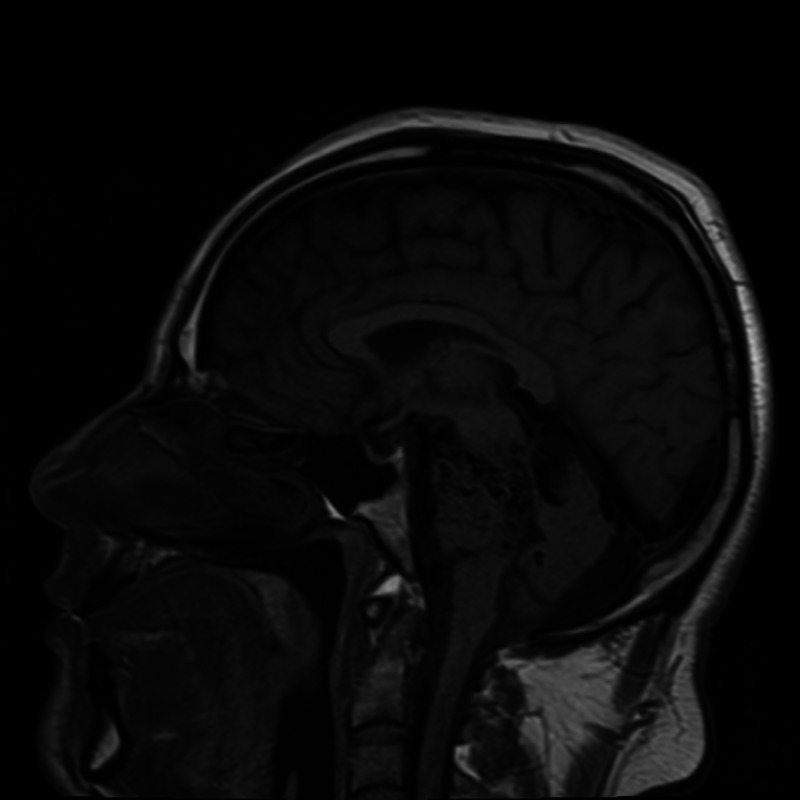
MRI head without contrast, sagittal section
